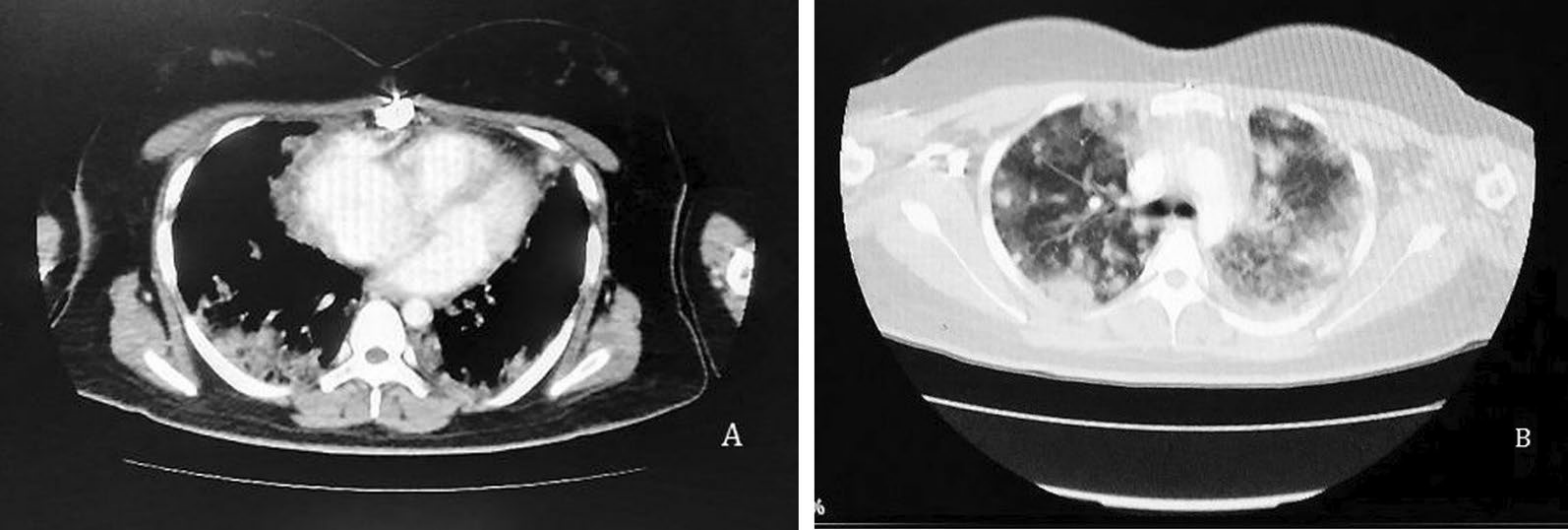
High-resolution computed tomography (HRCT) of the patient showing A mild pleural effusion with dilated left atrium and B bilateral pulmonary multi nodular consolidations
